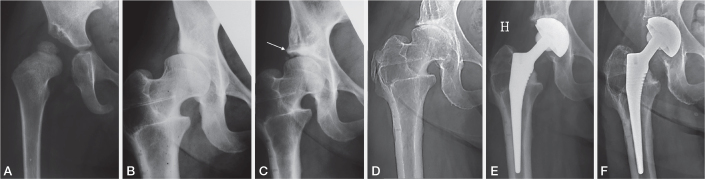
(A) Initial pelvic radiograph of a girl at the age of 2.7 years, showing a dislocated right hip. (B) The same patient at the age of 20 years shows residual dysplasia of the right hip. A varus femoral osteotomy had been performed at the age of 16 years. A modified Spitzy shelf arthroplasty was performed 10 months after this radiograph. (C) Radiograph 3 years after the Spitzy operation, showing improvement of the acetabular roof and satisfactory femoral head coverage. The shelf is indicated by an arrow. (D) Radiograph at the age of 48 years, showing osteoarthritis of the right hip with narrowing of the joint space. (E) Radiograph 3 months after total hip arthroplasty. (F) Last radiograph at patient age 69 years, showing no signs of loosening of the hip prosthesis.
